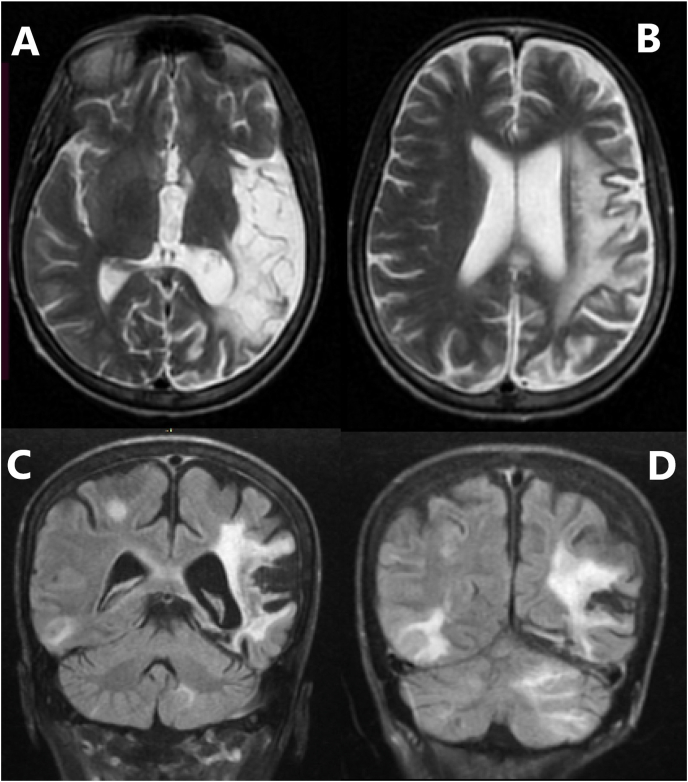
T2 weighted MRI: A; Axial T2 shows hyperintense signal in the left paretial and temporal lobes indicates an old infarction. B; Axial T2 shows hyperintense signal in the left paretial, temporal, occipital lobes C; Coronal T2 shows hyperintense signal in the left paretial and temporal lobes, atrophy of gray matter of the partial lobe shows as hypointense signal (arrow), tow foci of hyperintense-white matter lesions in paretial and temporal lobes. D; Coronal T2 shows hyperintense signal in the left paretial and temporal lobes, hyperintense-white matter lesion in right temporal lobe, hyperintense signal in left cerebellum.
